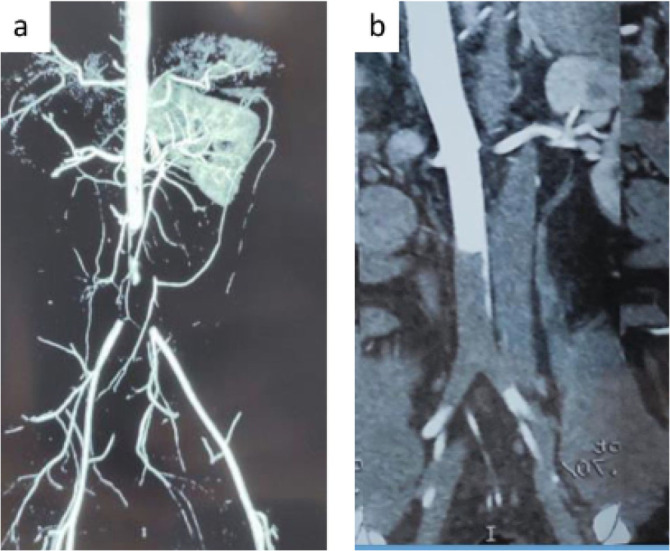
CT angiogram of aorta showing infra renal abdominal aorto-iliac thrombosis.
